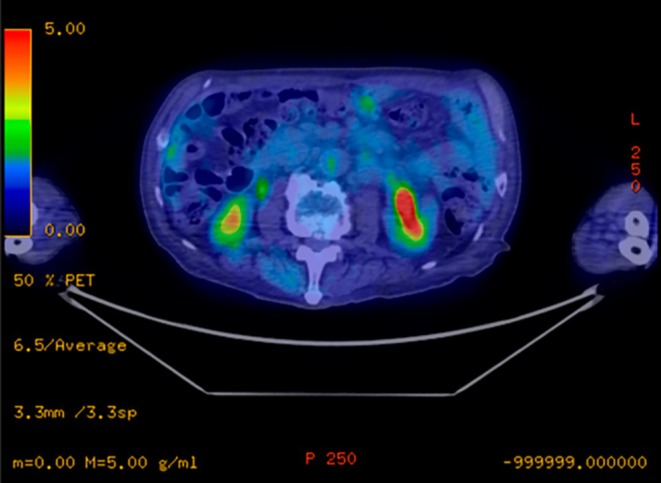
PET‐CT scan image showing no tracer accumulation in abdominal lymph nodes.
